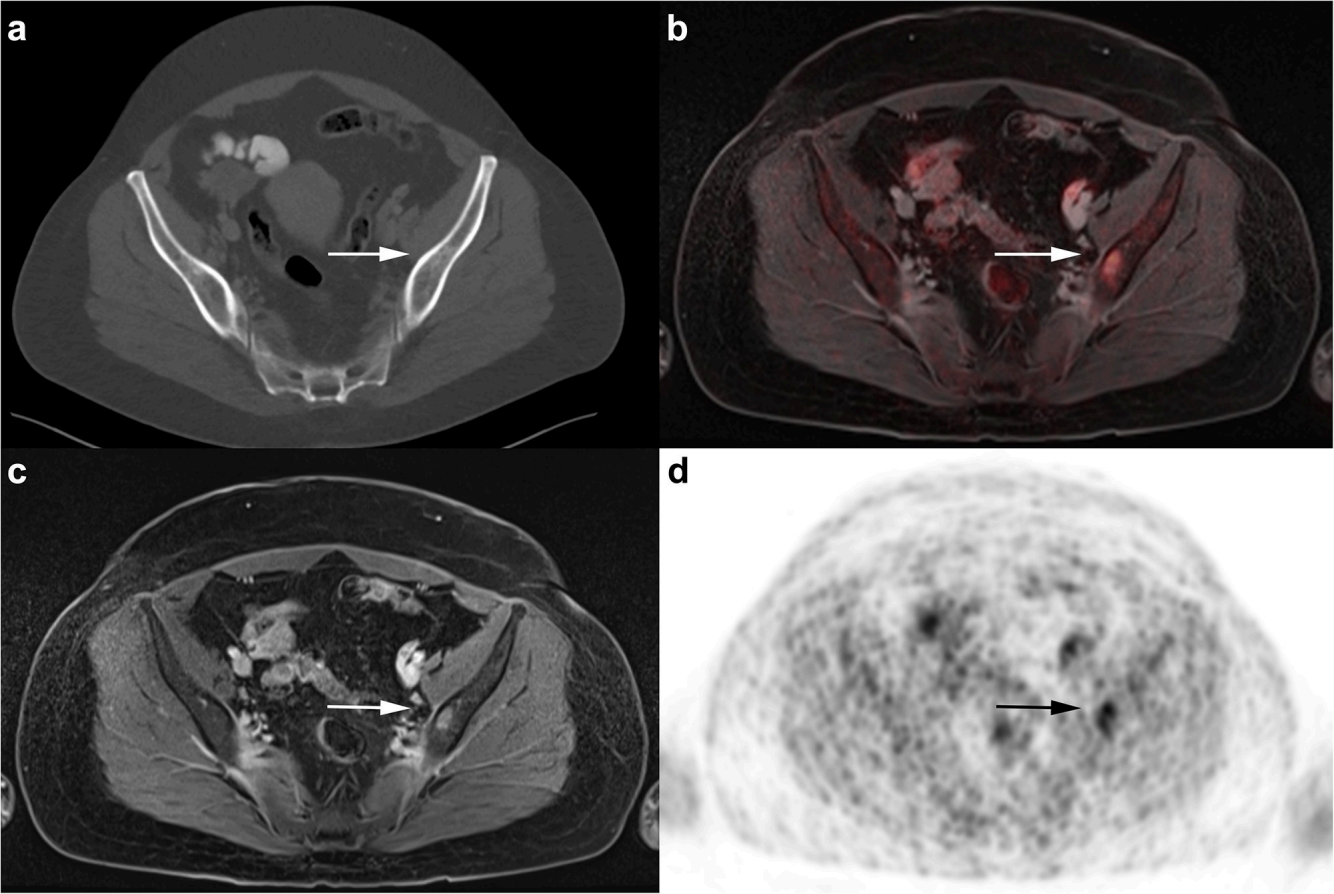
A 45-year old woman with diagnosis of primary breast cancer. No distant metastases were detected in the CT scan (a). The subsequently performed ^18^F-FDG PET/MRI shows a bone metastasis in the left iliac bone with contrast enhancement on T1w fs VIBE (c) and pathological FDG uptake on PET (d) and fused ^18^F-FDG PET/MRI (b).
